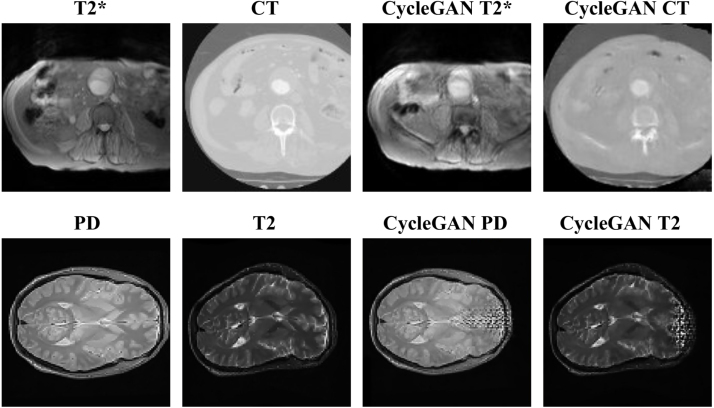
Example of cross-domain synthesis using vanilla CycleGAN. The first row shows the results obtained from cross-modality abdominal MR-CT data; the second row shows the results of multi-sequence brain data with a synthesized deformation. Both cases demonstrate a reproduction of “domain-specific deformation” in the synthesized output.
